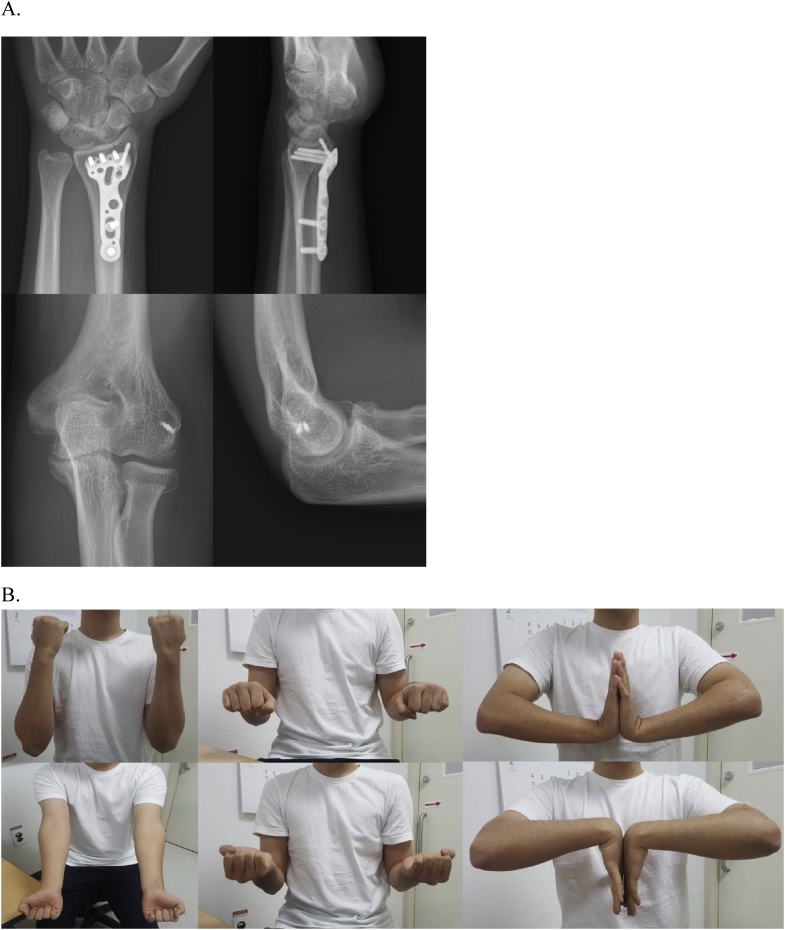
A, simple radiographs of left wrist and elbow at 1 year later after surgery demonstrate complete fracture union of distal radius and congruent elbow joint. B, Clinical pictures at final follow-up demonstrate full range of motion of both wrist and elbow joint.
